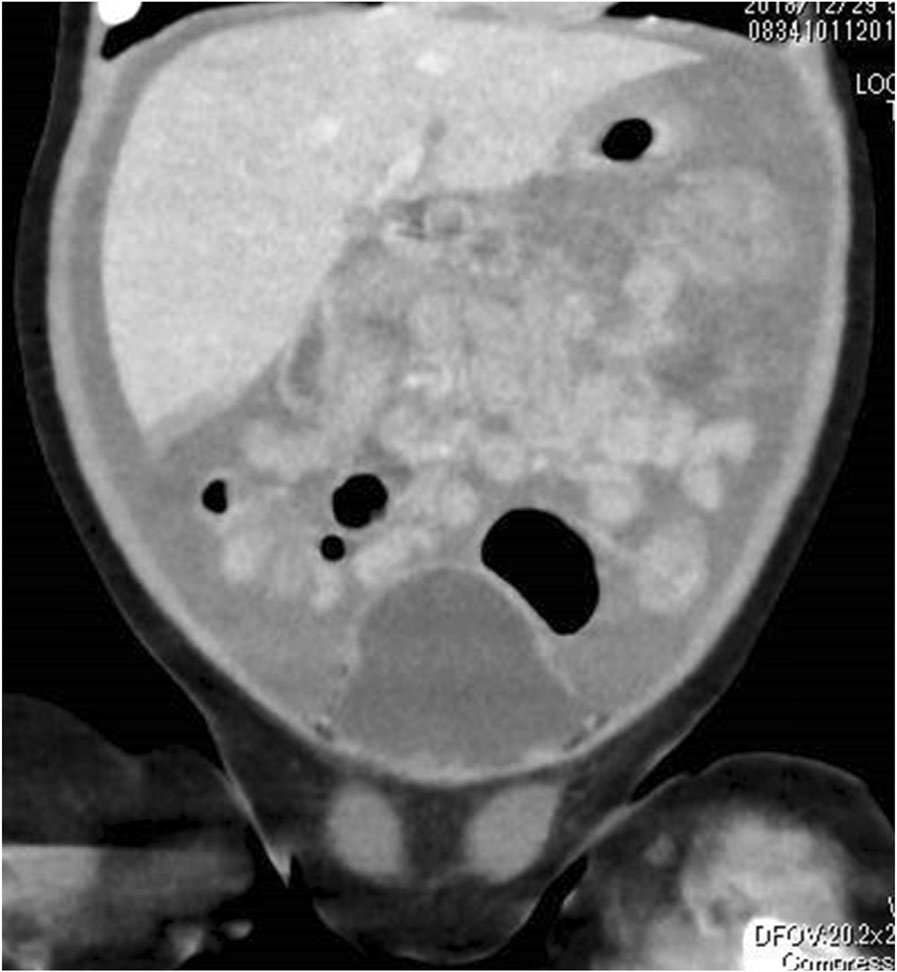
Computed tomography (CT), case 1. CT reveals intraperitoneal hemorrhage, but without extravasation
